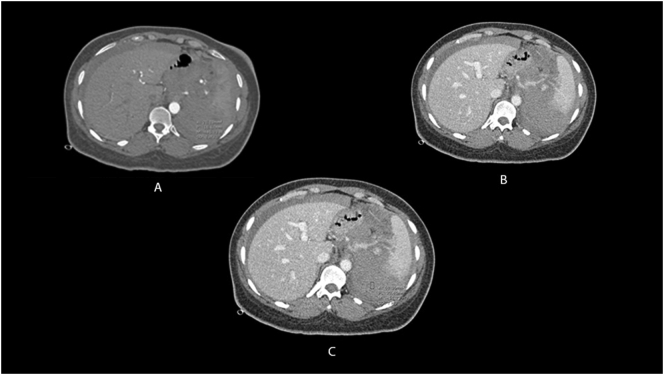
Abdominal CT angiogram arterial phase (a), venous phase (b&c).
